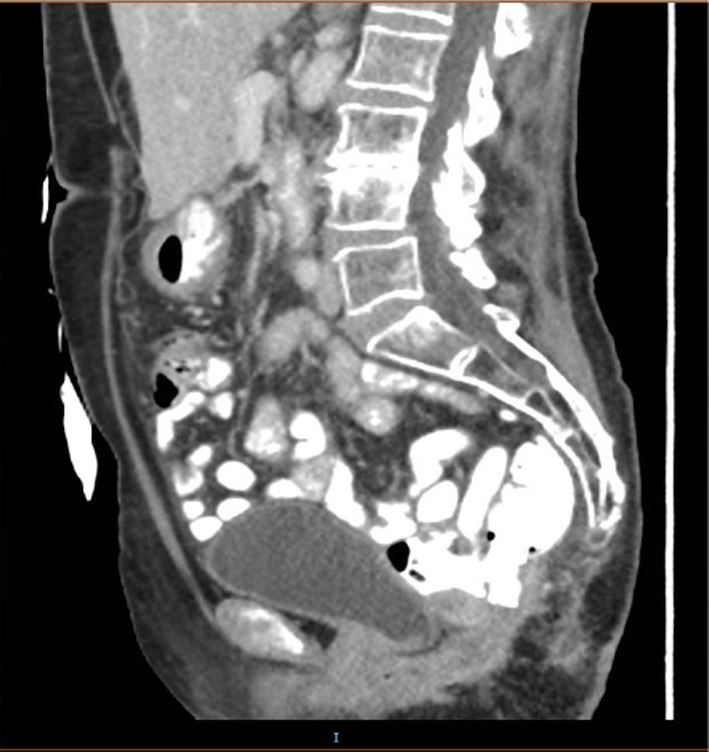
A follow up CT-scan showing results of the hernia repair, 2 months after the operation
